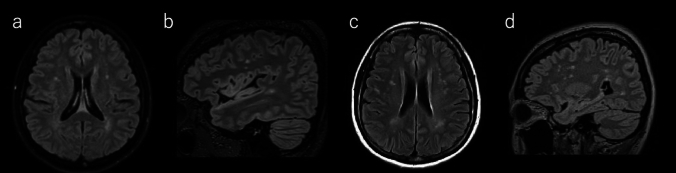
Representative case of multiple spotty lesions. Brain magnetic resonance images from case 8 at initial presentation (a, b) and at follow-up 6 years after onset (c, d). Axial Fluid-attenuated inversion recovery (FLAIR) images (a, c) and sagittal FLAIR images (b, d) demonstrate small, discrete white matter lesions located in the periventricular region but not abutting the lateral ventricles. Lesions remained non-confluent and radiologically atypical over time
